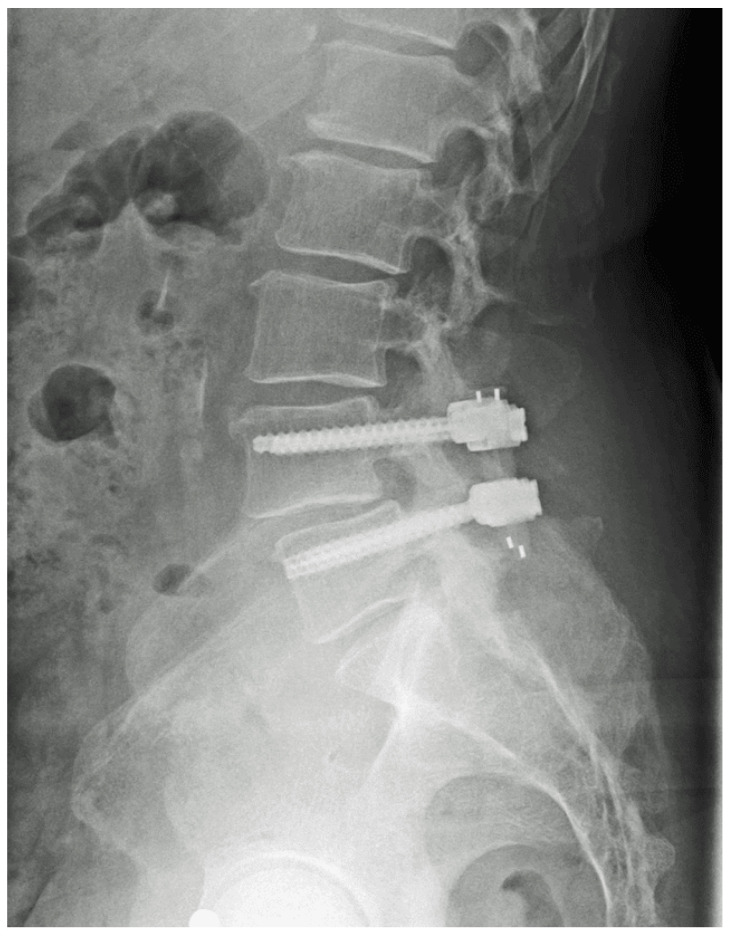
Postoperative X-ray after decompression and instrumentation at L4/5. Images are published under a Creative Commons license [1].
